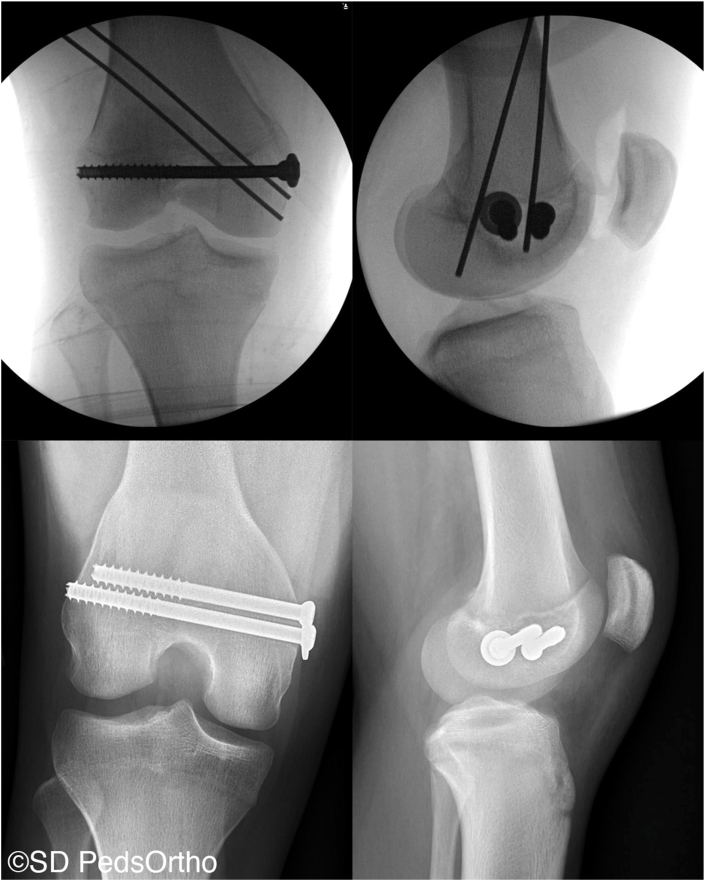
Intraoperative fluoroscopy (top) and postoperative radiographs (bottom).
